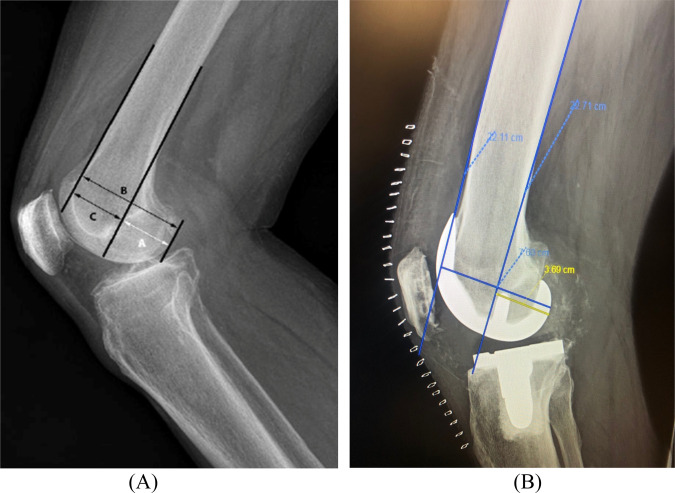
A, Radiographic determination of PCOR. PCOR is defined as the ratio of the posterior condyle offset to the diameter of the femur, 2.5 cm above the termination of the supracondylar flare on true lateral view. The first line is drawn tangential to the anterior femoral cortex, and a second line is placed parallel to the first line, tangential to the posterior femoral cortex. A third line is placed parallel to the first two lines, along the posterior aspect of the posterior femoral condyle. “A” represents the PCO, “B” represents the distance from the first line to the third line, and “C” represents the distance from the anterior cortex of the femur to the posterior cortex of the femur. PCOR is defined as A/B. B, Radiographic determination of PCOR in a TKA. PCO = posterior condylar offset, PCOR = posterior condylar offset ratio, TKA = total knee arthroplasty
